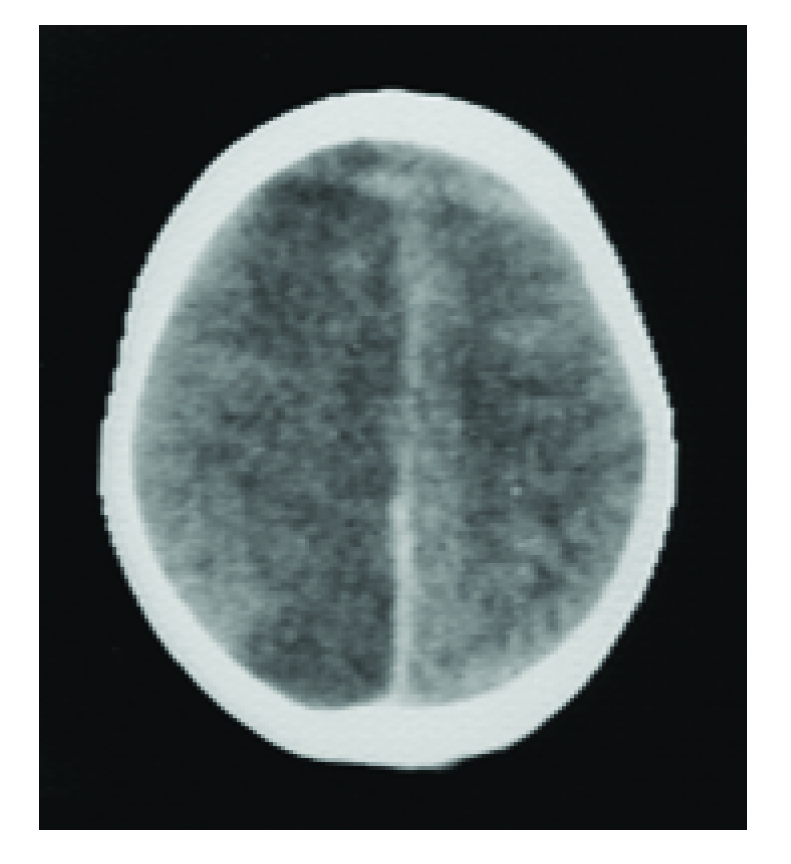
Diffuse brain swelling. CT–scan shows extensive diffuse ischemia (‘black brain’).
